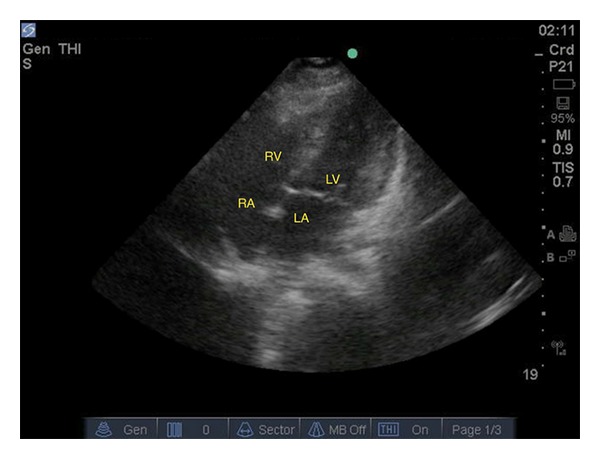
Apical four-chamber view showing normal RV : LV ratio.
